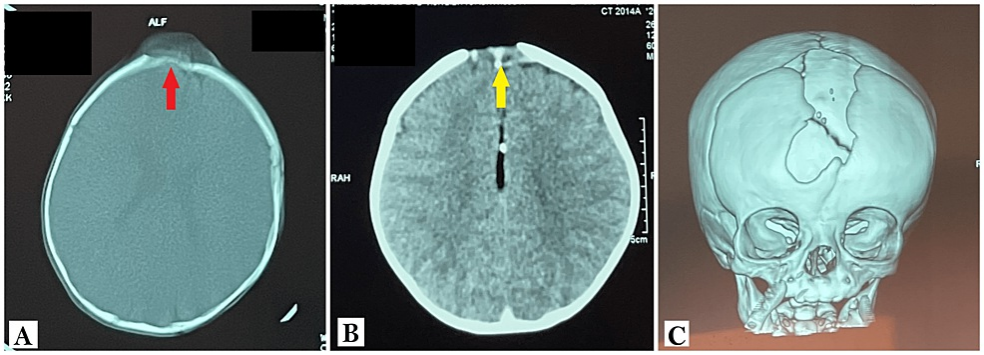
CT scan of the head showing the frontal mass. A: CT scan of the head in the bone window, in an axial plane, showing a well-defined isodense frontal mass (red arrow), with an invasion of the underlying bone resulting in a bony defect. B: CT scan of the head in the brain window, in an axial plane, showing the same lesion (yellow arrow) with no extension to the parenchyma and normal-looking lateral ventricles. C: CT scans 3D reconstruction showing the extent of the bony defect.
